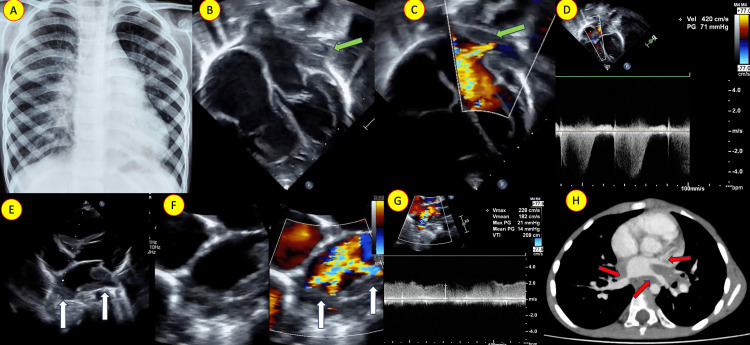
Pulmonary vein obstruction A: Chest X-ray (posterior-anterior view) indicating pulmonary venous congestion. B, C: Echocardiogram in four-chamber view showcasing dilated right heart chambers and obstruction in the left pulmonary vein by external tissue (green arrow). D: Continuous wave Doppler of the tricuspid regurgitation jet demonstrates elevated right ventricular systolic pressure. E, F: Parasternal short-axis view with color Doppler displaying obstructed pulmonary veins by soft tissue (white arrow). G: Doppler shows a significantly elevated mean gradient across the pulmonary vein. H: Computed tomography angiography depictis diffuse soft tissue (red arrow) in the mediastinum encasing the pulmonary veins, causing obstructions.
